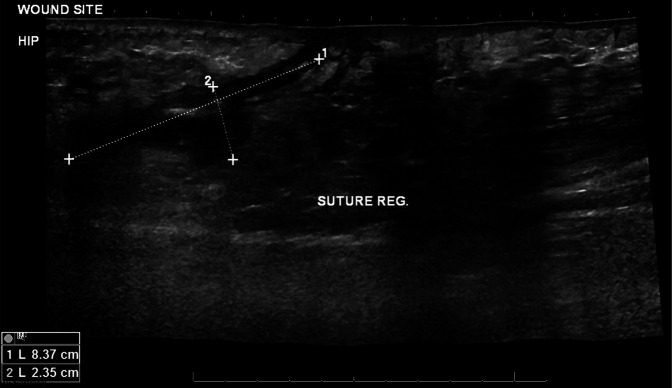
Ultrasonography of the lateral soft-tissue of the hip showing a subcutaneous collection measuring 8.4 cm by 2.4 cm. This collection was used as an indirect indication of the presence of OM because it is likely to have formed secondary to the infection.
